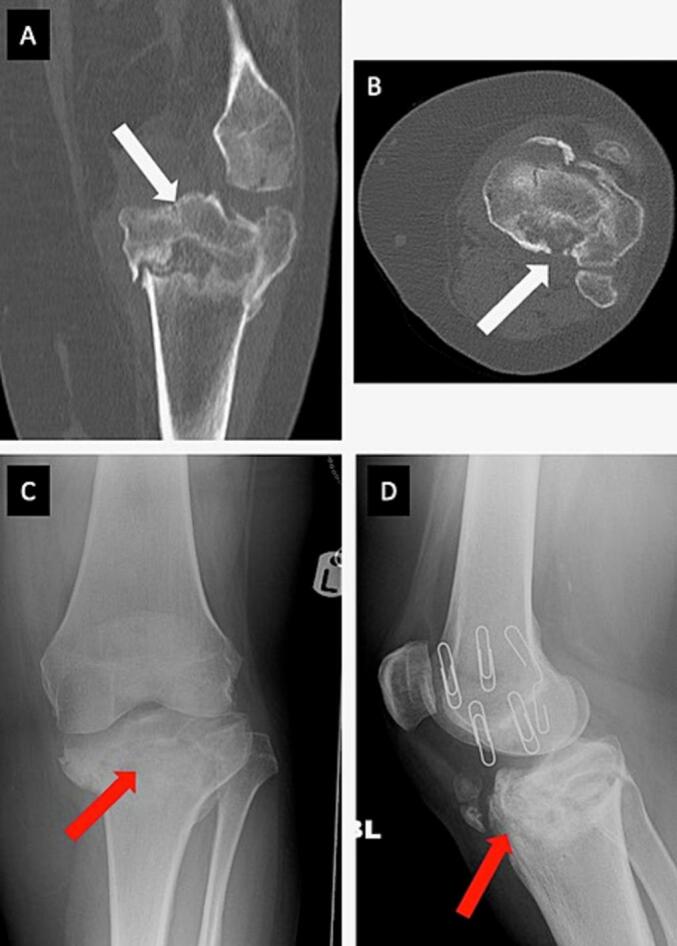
A & B) CT imaging of the left knee joint demonstrating fracture union of the left tibial plateau (white arrows); C & D) X-ray images obtained at initial presentation to our centre. These radiographs demonstrate (red arrows) a non-united bicondylar tibial plateau fracture in hyperextension and a varus position. (For interpretation of the references to colour in this figure legend, the reader is referred to the web version of this article.)
